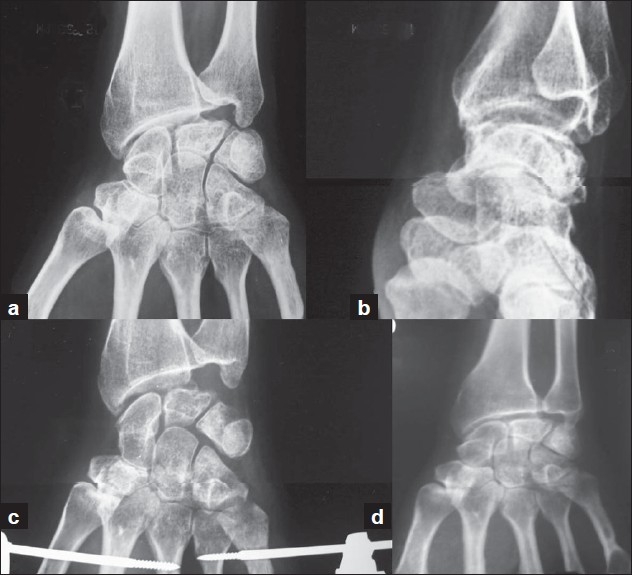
Preoperative X-rays of the wrist left side [Anteroposterior (a)and lateral (b) views] of case III with Kienbock's disease (stage II) showing normal outline of lunate with density changes. Postoperative anteroposterior views of the wrist of the same case at 8 weeks. (c) Shows maintained outline with reduced density compared to preoperative X-ray. 5 years follow-up X-rays. (d) Shows maintained carpal height with no degenerative changes
